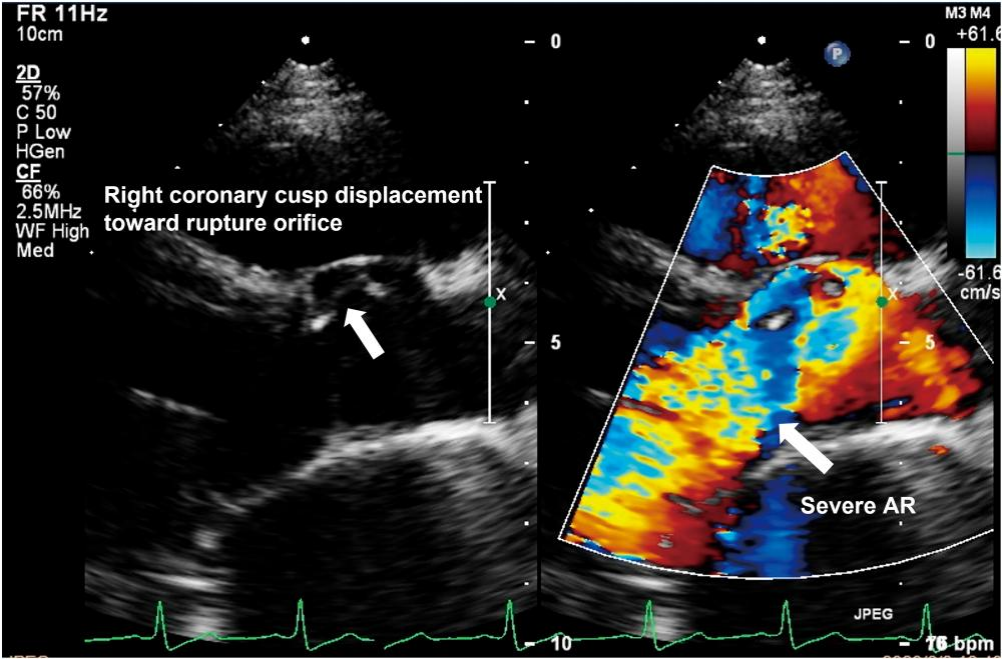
Parasternal long-axis TTE. (*A*) Diastolic malcoaptation with the right coronary cusp held open (white arrow). (*B*) Colour Doppler shows severe aortic regurgitation (white arrow).
